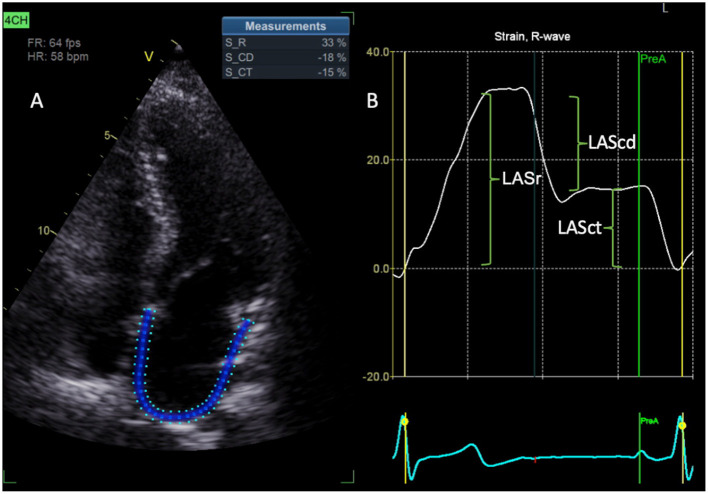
LAS assessment using 2D speckle-tracking echocardiography in a representative patient who presented with AIS. (A) Apical 4-chamber view with left atrial endocardial border tracings. (B) R-R gated LAS curve with annotations depicting the three phases of the LA cycle: LASr, LAScd, and LASct. AIS, acute ischemic stroke; LAS, left atrial strain; LAScd, left atrial conduit strain; LASct, left atrial contractile strain; LASr, left atrial reservoir strain.
